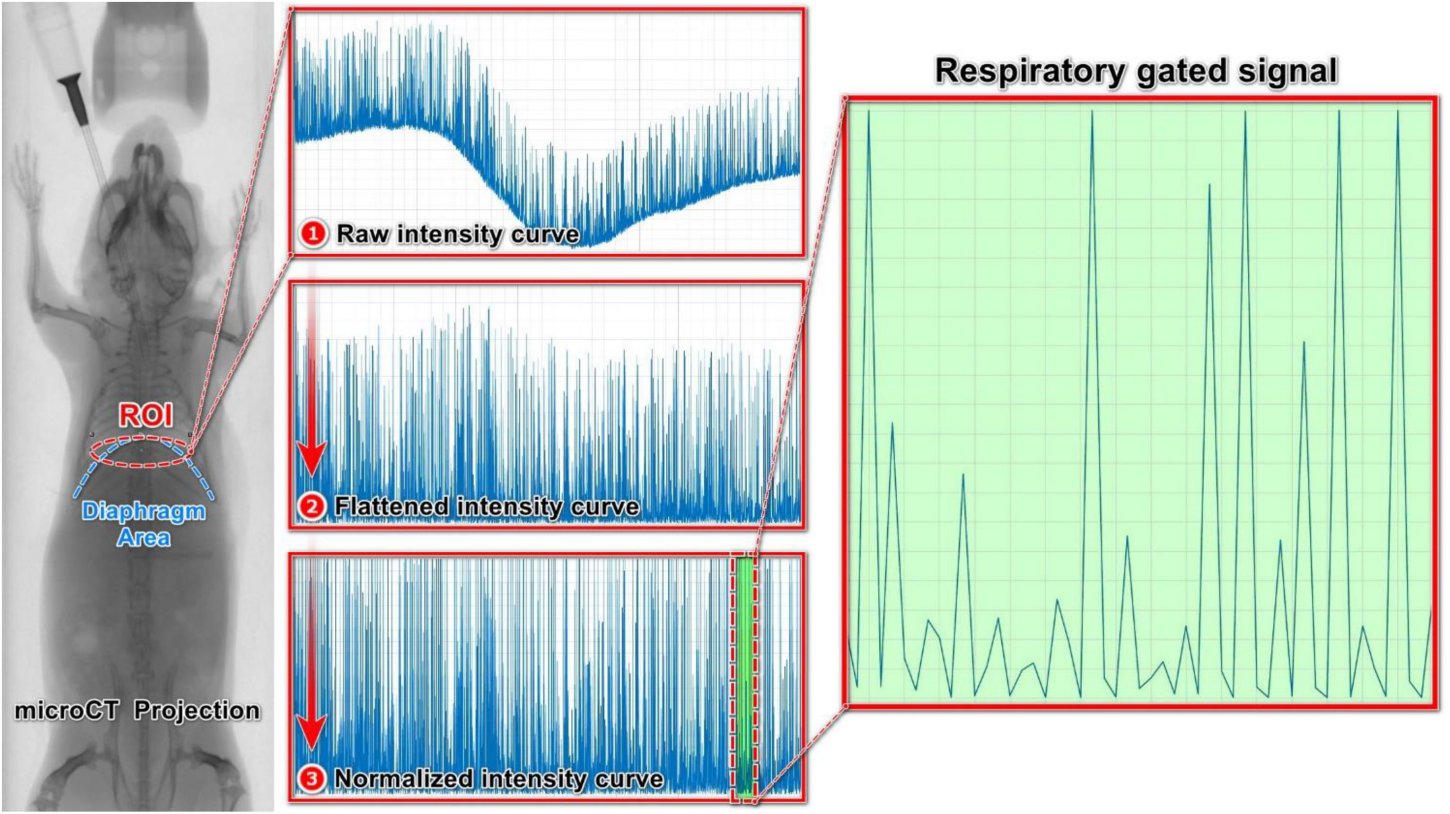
Extracting respiratory signal from a raw intensity curve using respiratory gating technique: (1) Raw intensity curve obtained from X-ray projections. (2) Flattened intensity curve, by subtracting the baseline of the raw intensity curve. (3) Normalized intensity curve, normalized by the local maximum of each angular position.
